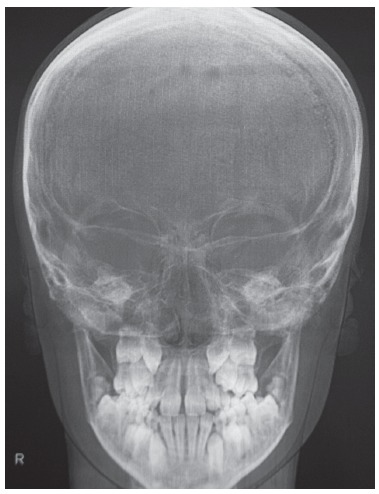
PA cephalometric radiograph pre-RME.
